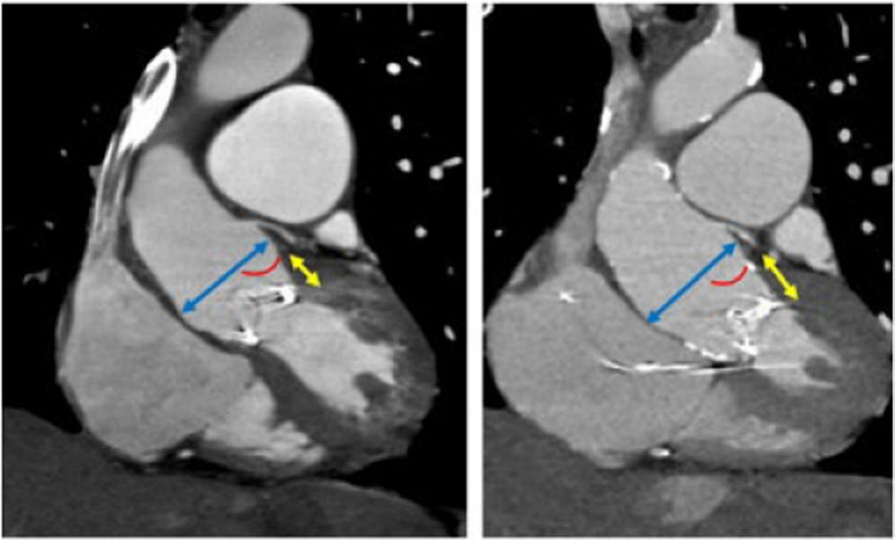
Computed tomography images revealed the malposition of the bioprosthesis, and gradual increase in diameter between the original annulus line and the bioprosthesis. (Rt) 1 year after the initial surgery, (Lt) 13 years after the initial surgery
